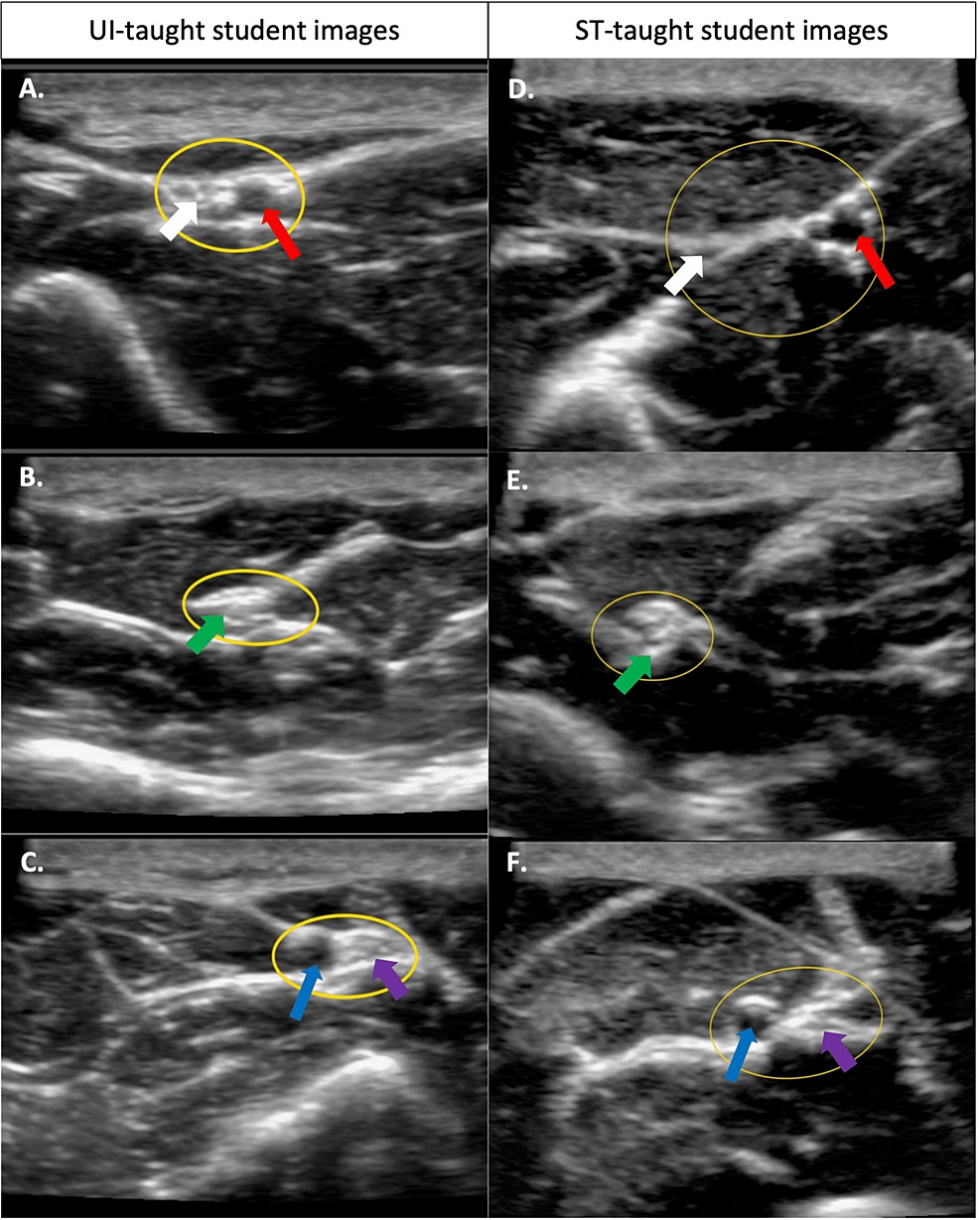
Comparison of ultrasound image quality taken by students taught by UIs or STs. A, B, and C were imaged by the UI group, and D, E, and F were imaged by the ST group. Yellow circle (part of the original image) = made by students to identify nerves and arteries; white arrow = radial nerve; red arrow = radial artery; green arrow = median nerve; blue arrow = ulnar artery; purple arrow = ulnar nerve; ST = student tutor; UI = ultrasound instructor
